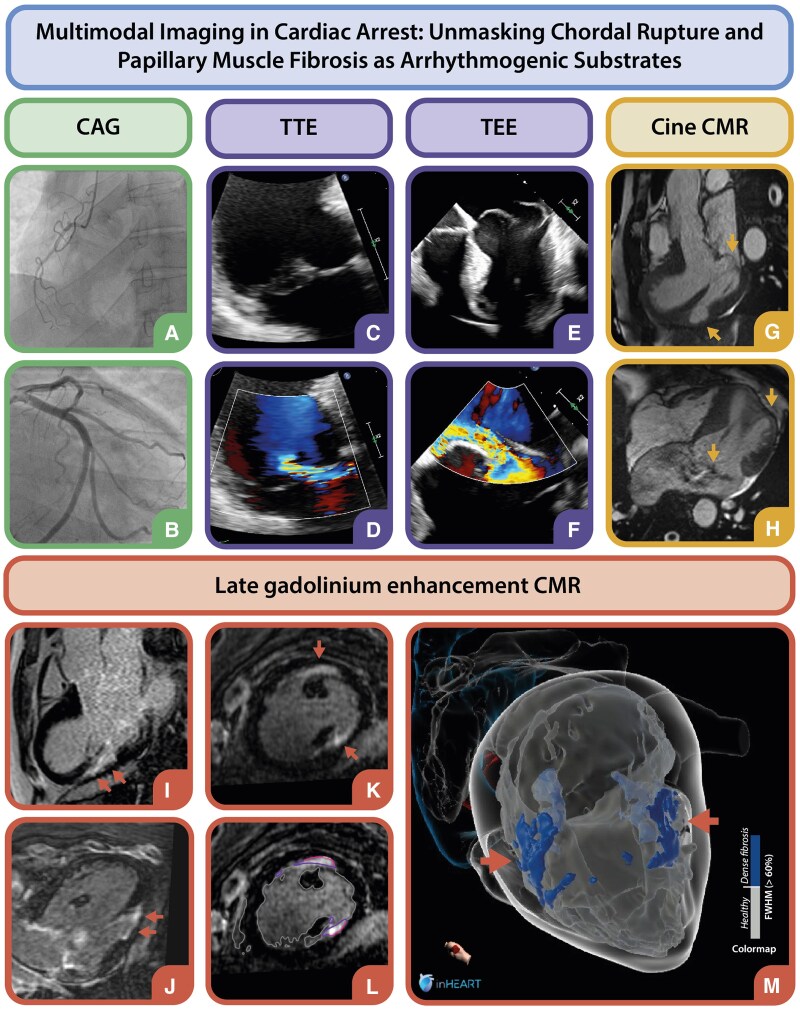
A 53-year-old male, previously evaluated for paroxysmal atrial fibrillation and mitral valve (MV) prolapse, was admitted after a witnessed out-of-hospital cardiac arrest due to ventricular fibrillation (VF). Paramedics delivered eight shocks for recurrent VF. Initial workup showed normal electrolytes and no evidence of ischaemia or genetic channelopathy. Coronary angiography excluded significant coronary artery disease (CAD) (*Panels A* and *B*). Transthoracic echocardiography, however, revealed a dilated left ventricle (LV) with mild dysfunction and severe mitral regurgitation (MR) due to posterior leaflet flail (*Panels C* and *D*). Trans-oesophageal echocardiography confirmed bi-leaflet myxomatous Barlow’s disease with anterior leaflet prolapse and posteromedial papillary muscle chordal rupture (*Panels E* and *F*).
